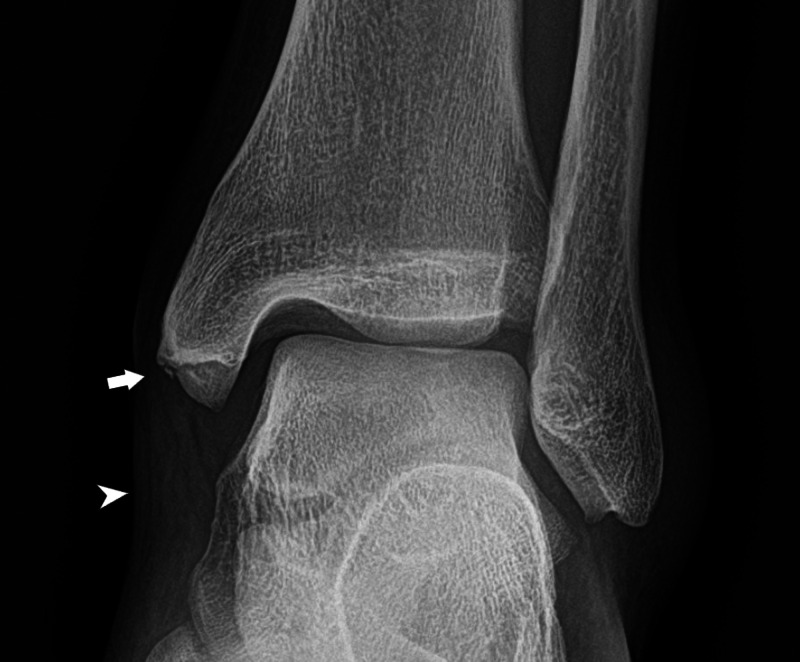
Case 3 ankle radiograph A frontal radiograph of the ankle demonstrates a small avulsion fracture of the medial malleolus (white arrow) with moderate soft tissue swelling (white arrowhead).
